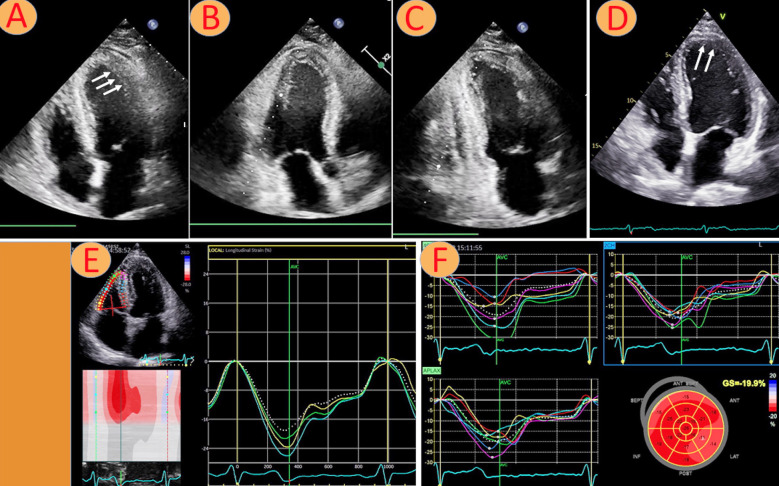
Echocardiographic findings of edema of the Left ventricle (A,B arrows). Significant improvement of left ventricular thickness (C, D arrows). Normalization of right and left ventricular global longitudinal strain (GLS) at 2 month follow-up (E-F).
